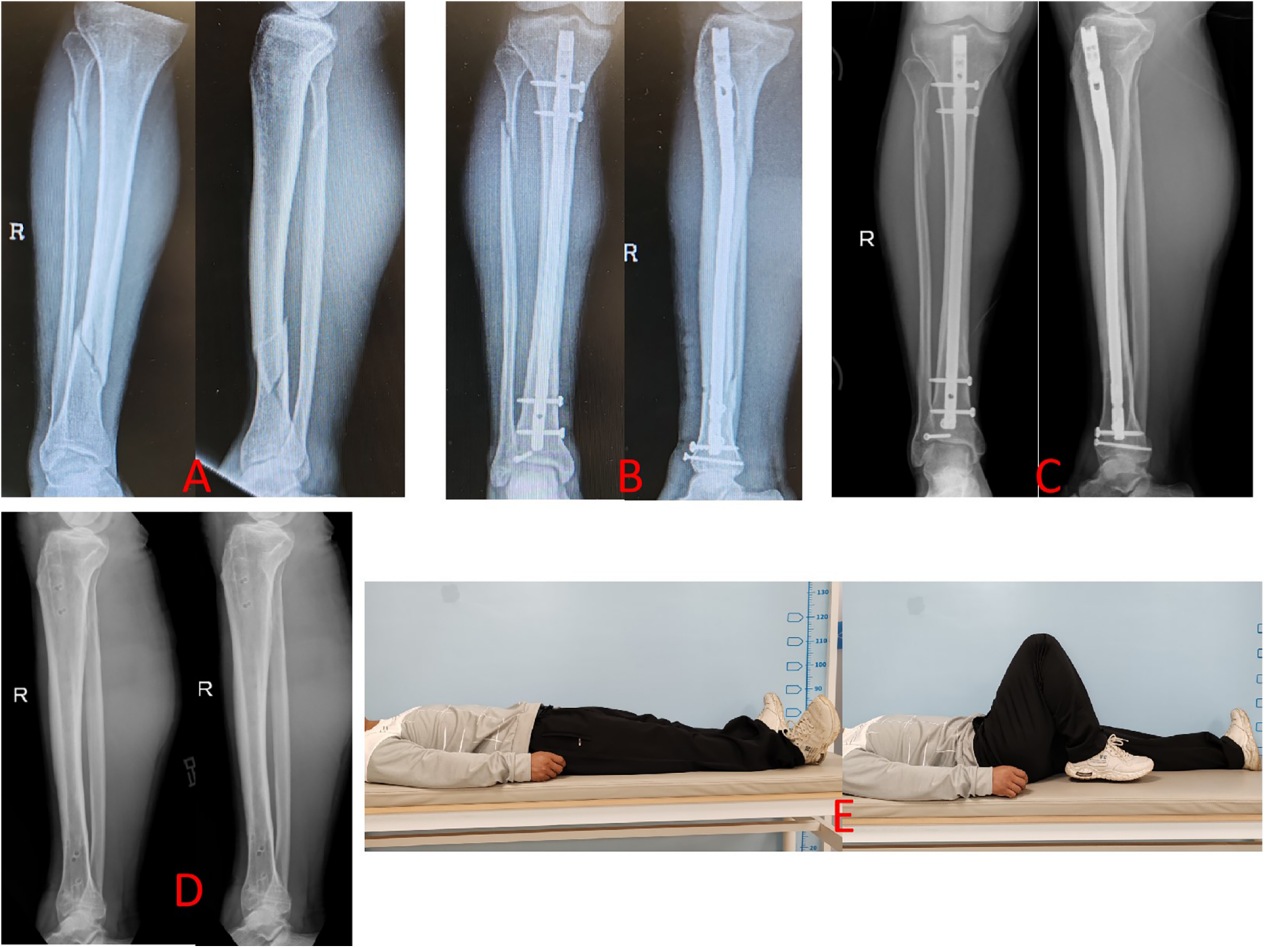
Radiographs of a case (a 29-year old man with the right tibia shaft fracture (AO- 42B3) of union after closed reduction using the IP approach were presented. (A) Preoperative anteroposterior (AP) and lateral views. (B) AP and lateral views postoperatively. (C) AP and lateral views 12 months postoperatively. (D) AP and lateral views after IMN was removed. (E) Functional recovery at 12 months postoperatively.
